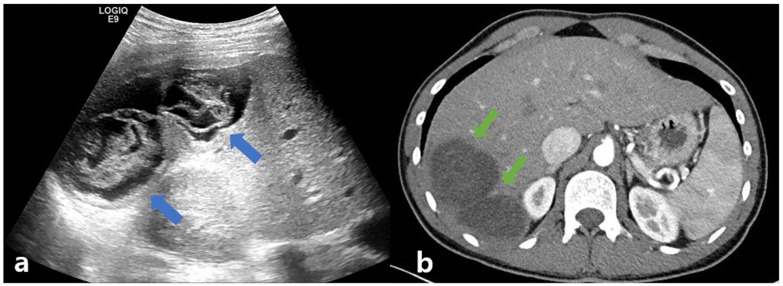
Abdominal ultrasonography (a): two communicating, incomplete loculated anechoic lesions, measuring approximately 8–11 cm, filled with folded linear echoic materials (blue arrows). Abdominal computed tomography (b): two communicating hypodense cystic lesions, measuring approximately 8–11 cm, filled with slightly enhancing material (green arrow).
